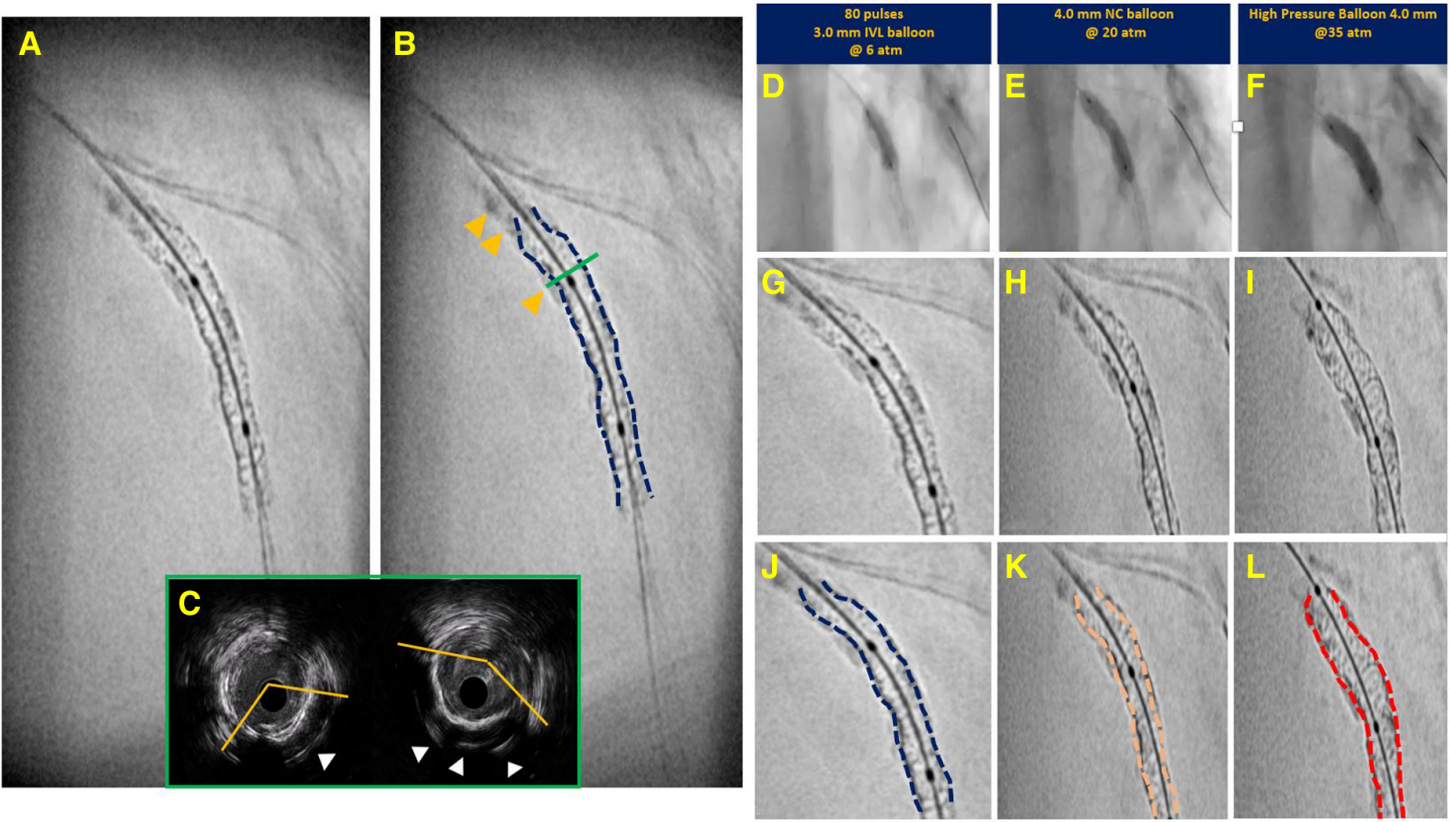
Case example of calcified in stent restenosis. Enhanced angiography (Panel A) proved helpful in defining presence of significant calcified component (yellow arrowhead in Panel B) and associated stent underexpansion (dotted blue line). On Intravascular ultrasound (at the level of green line in Panel C), calcium component appeared not circumferential and possibly thin according to evidence of reverberations (white arrowheads), even though they could have been misinterpreted for stent struts). Panels D,E show resistant calcium with underexpansion of intravascular lithotripsy (IVL) and non-compliant (NC) balloons. Eventually expansion achieved with high pressure balloon (Panel F). Comparing enhanced angiography at each step (baseline—Panel G–J; after lithotripsy and NC dilation in Panels H–K; after high pressure balloon in Panels I–L) it is possible to appreciate degree of calcium modification with increasing improvement of stent expansion.
